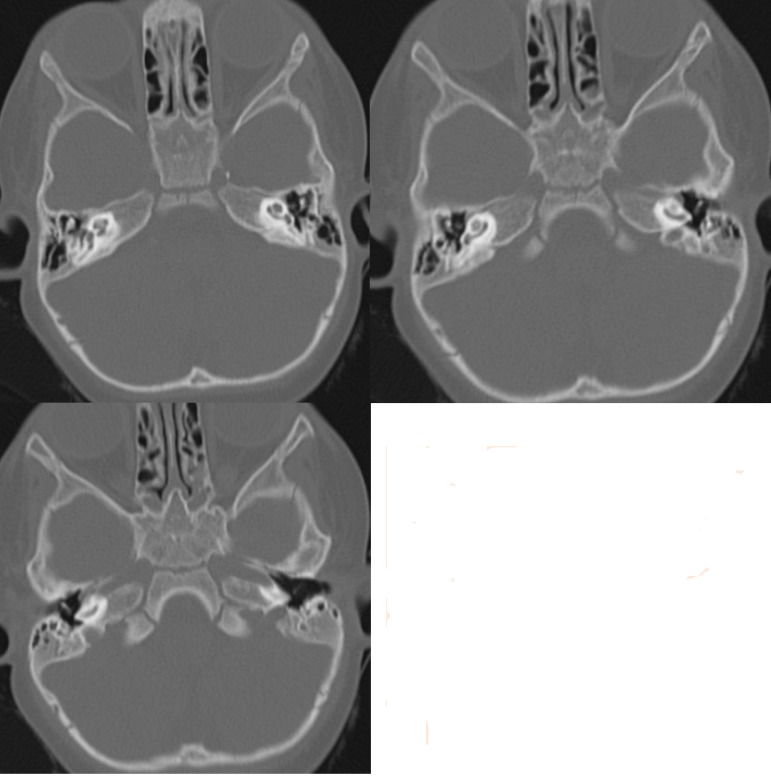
Mastoid CT scan of the patient with meningitis in his history
